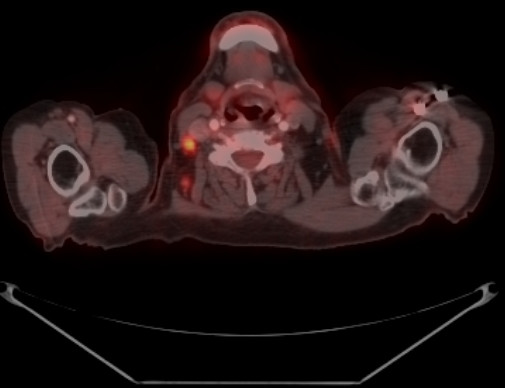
Transverse slice demonstrating FDG-positive lymph node. An US-guided FNA of this node was non-diagnostic.
